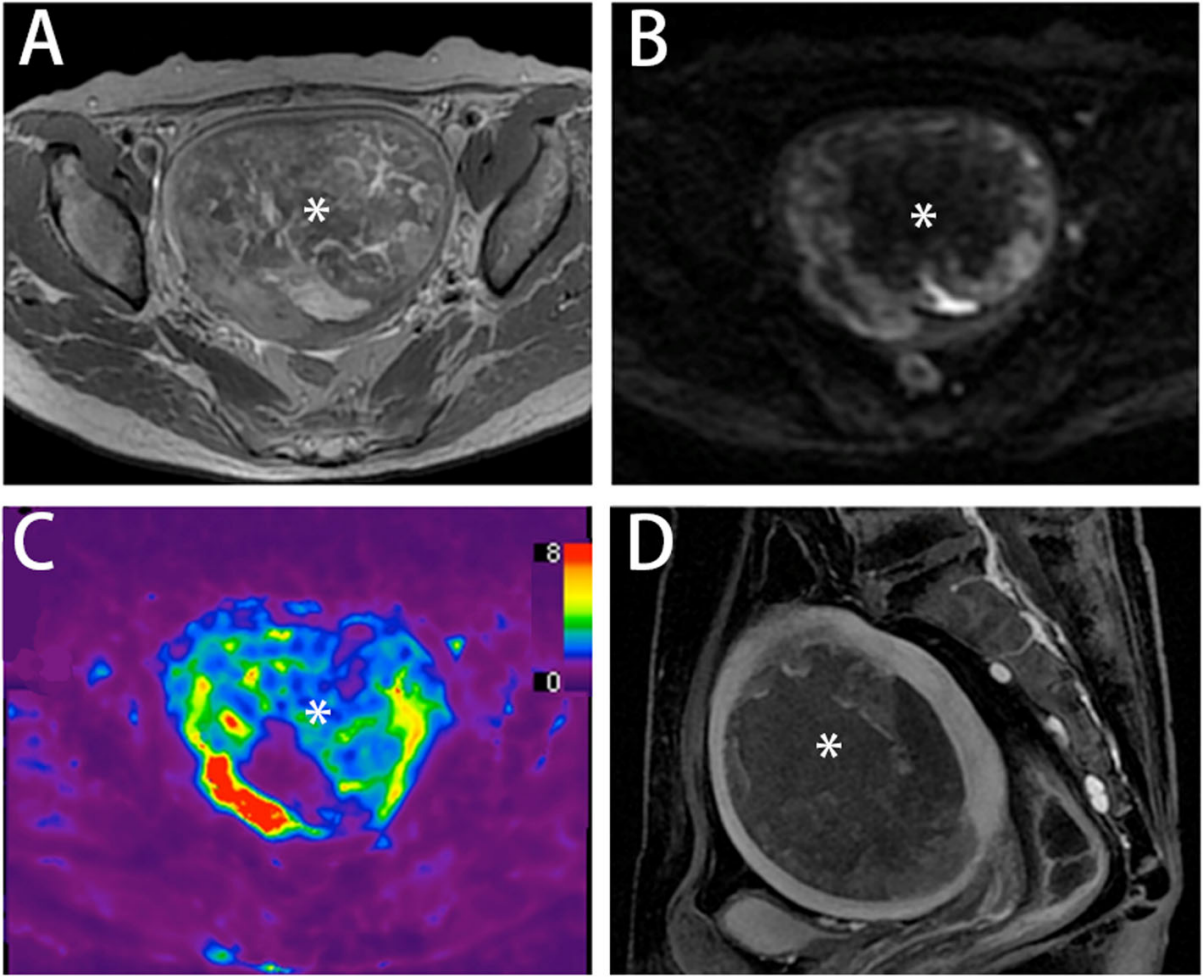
A 61-year-old woman presented with lower abdominal pain and underwent pelvic MRI and MRE scans. Axial T2-weighted image (A), diffusion-weighted image (B), and sagittal T1-weighted image with contrast (D) showed a mass (6.1×4.2 cm, asterisk) in the uterus. Tumor stiffness was 3.38 kPa measured by 3D MRE elastograms (C) at 60 Hz. Poorly differentiated endometrial adenocarcinoma (Type I) with deep myometrial invasion was surgically confirmed
